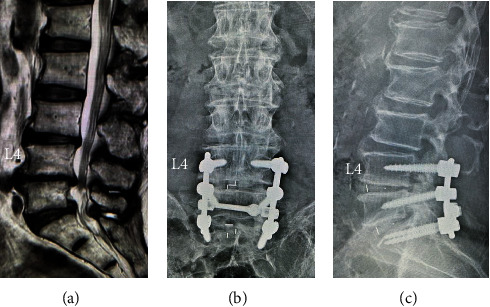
(a) Preoperative sagittal T2-weighed magnetic resonance imaging showing bulging discs and canal stenosis at L4-5 and L5-S1. (b, c) Postoperative radiographs after posterior fusion surgery at L4-S1.
